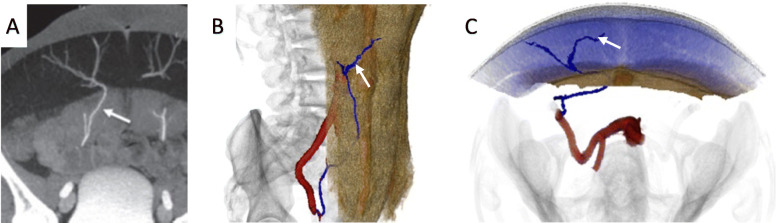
a Axial Maximum intensity Projection (MIP) image from a CTA demonstrating the deep inferior epigastric perforating vessels (arrow). b Coronal and c axial projections of a 3D rendering obtained from the CTA images showing the subfascial intramuscular course of the vascular tree
